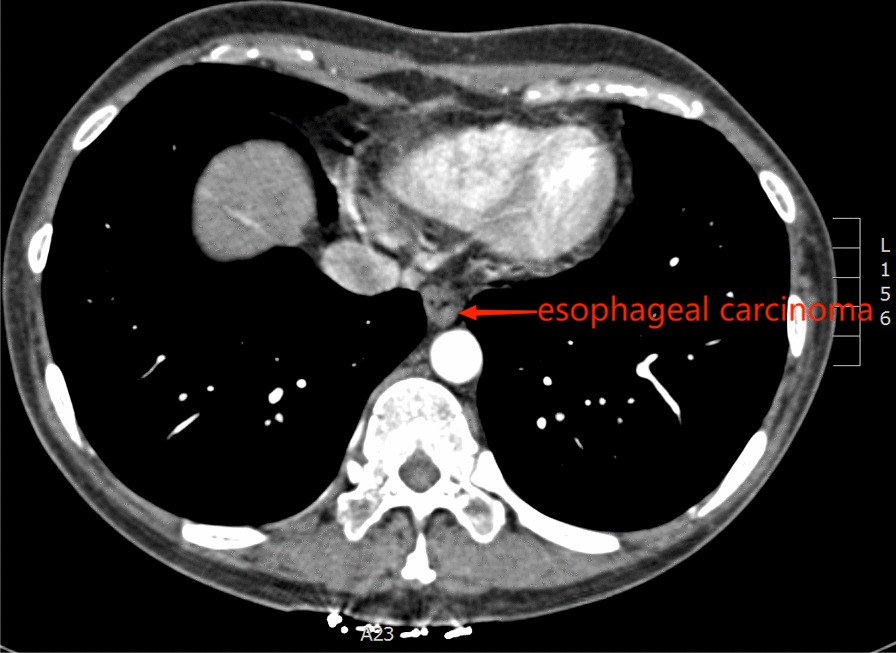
Chest CT before the operation of esophageal carcinoma
